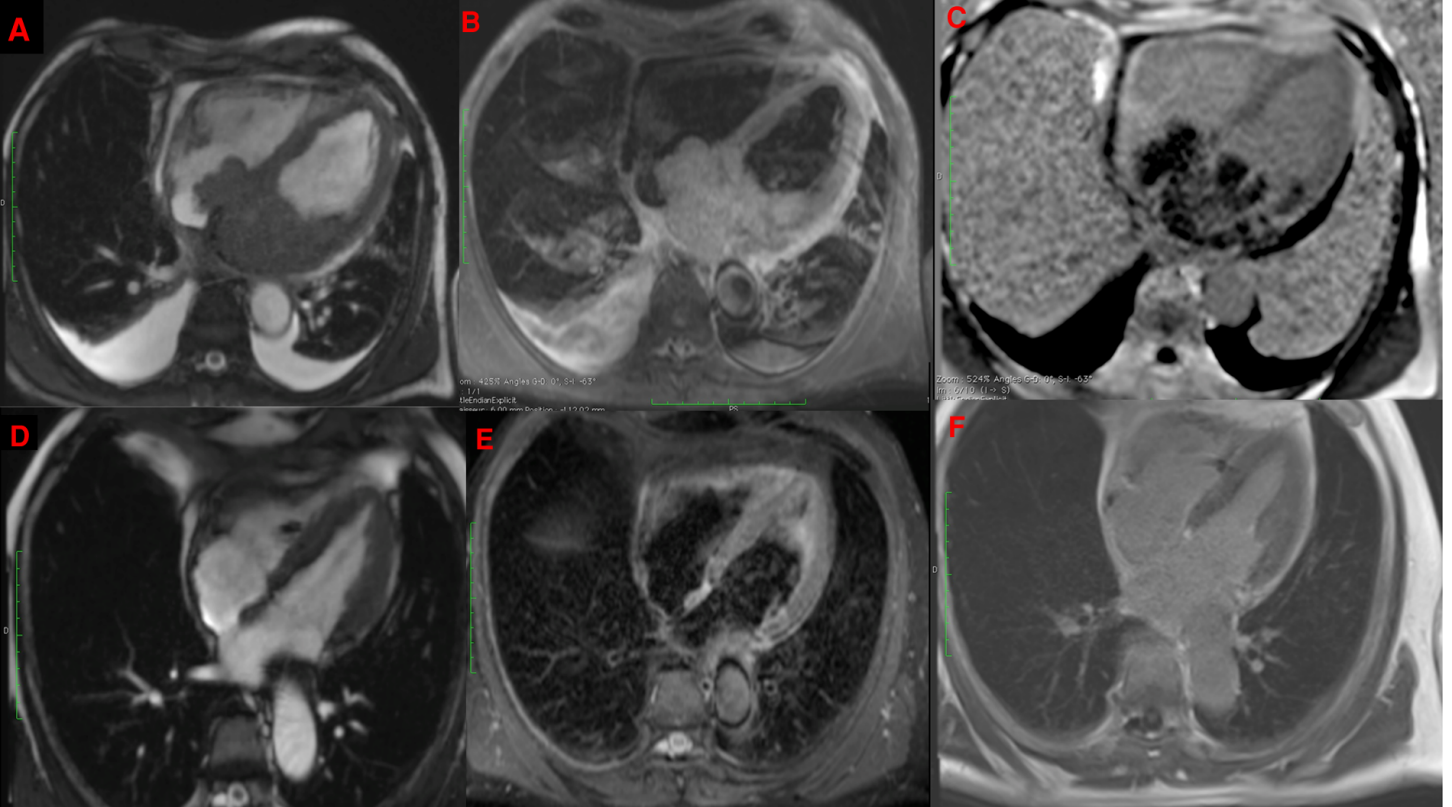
CMR showing a bulky mass extending throughout the left atrium, infiltrating the interatrial septum and right atrium, on cine imaging sequence (A), slightly hyperintense on fat-saturated T2-weighted imaging (B) with heterogeneous enhancement on late gadolinium enhancement sequences (C) with complete disappearance after 6 cycles of R-CHOP in the same sequences (D–F).
